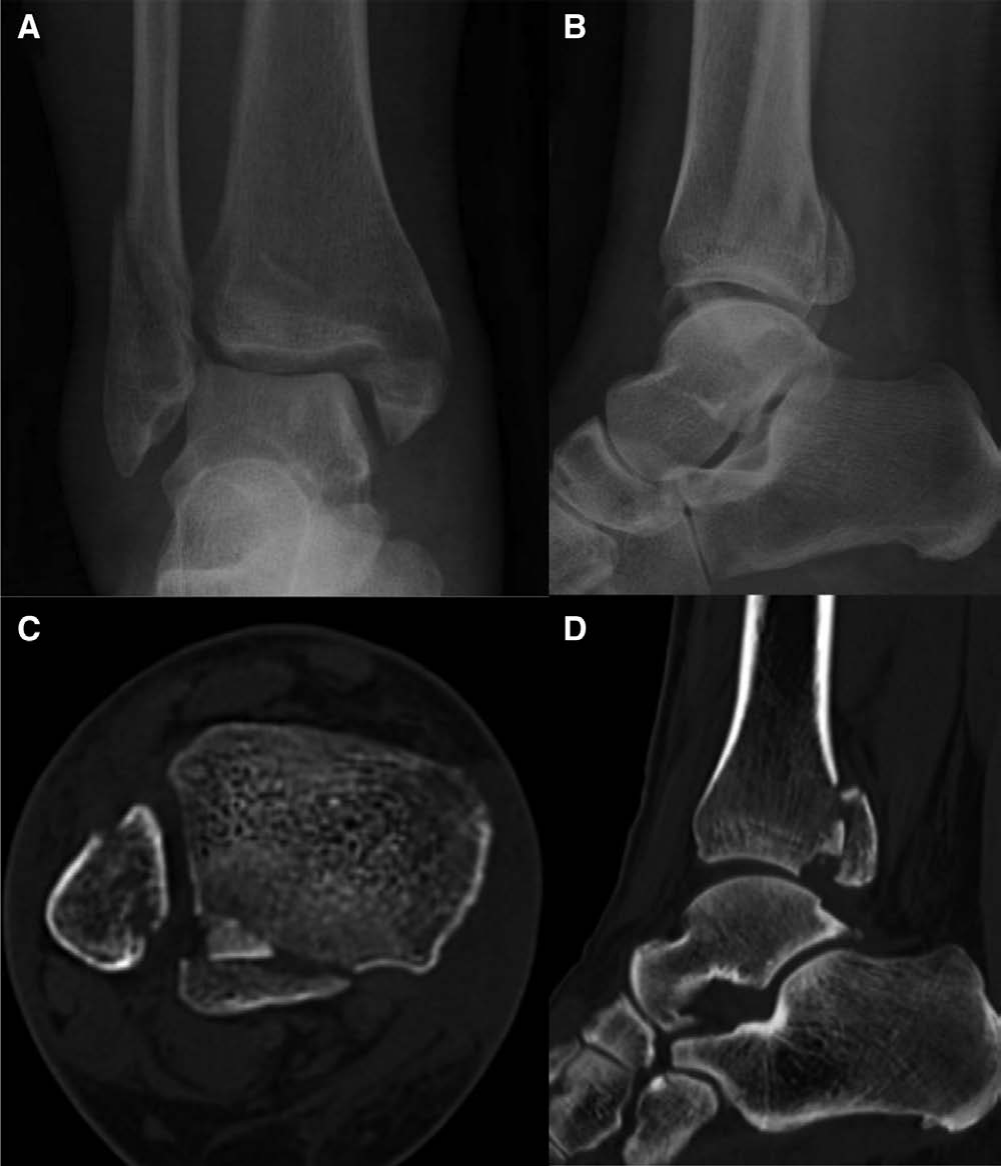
Pre-operative radiographs of the 52-year-old female patient with a trimalleolar fracture. (A) Anteroposterior and (B) lateral ankle plain radiographs. (C) Axial and (D) sagittal CT scans. An intra-articular incarcerated fragment was confirmed on CT. CT = computed tomography.
